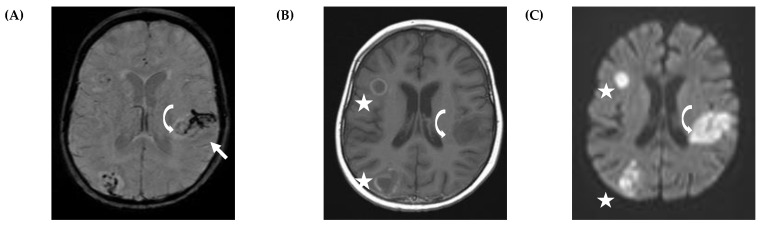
Vascular involvement in a 3-year-old girl with acute lymphoblastic leukemia (ALL) and proven central nervous system invasive mold infection (CNS IMD). The patient is alive but is a wheelchair user. Immunocompromised children have an impaired host immune system to fight against fungi (Figure 4A, curved arrow), which often results in an infiltration of the vascular structures (Figure 4A, straight arrow). Susceptibility-weighted image (SWI) (A) shows ring-like fungal infection on the left side (curved arrow) and thrombosis of the deep medullary veins (straight arrow), which are visualized as low signal intensity at SWI. Enhanced T1W (B) shows multiple ring-enhancing fungal abscesses on the right (star), but no enhancement on the left side. Diffusion-weighted image (DWI) (C) shows restricted diffusion within multiple ring-enhancing fungal abscesses on the right (star). The infarct with diffusion restriction (C) on the left involves the nonenhancing ring-like structure shown in the SWI (A, curved arrow).
